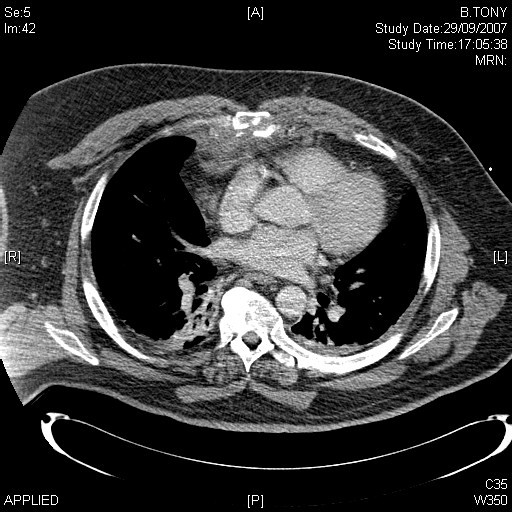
CT scan showing a comminuted fracture of the sternum and retrosternal haematoma.
